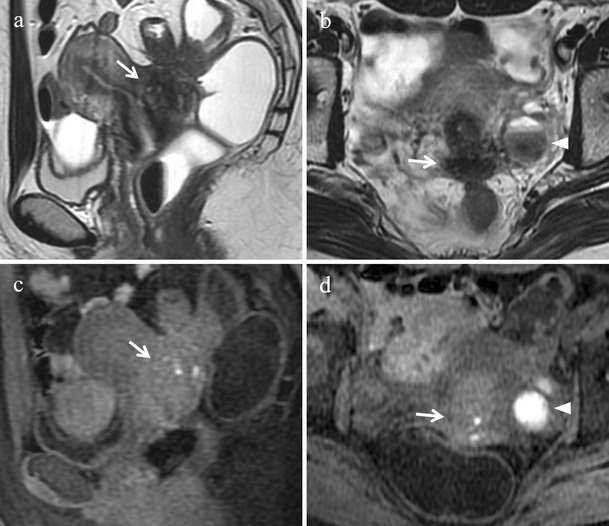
Endometriosis of the retrocervical region in a 41-year-old woman with dysmenorrhea and dyspareunia, who underwent previous laparoscopic adhesiolysis. (a) Sagittal and (b) oblique axial T2-weighted images show a hypointense ill-defined infiltrative tissue (white arrows) involving the posterior portion of the cervix, the retrocervical region and the anterior rectal wall. Small cystic cavities are seen within the lesion. On (c) sagittal and (d) axial fat-suppressed T1-weighted images the lesion exhibits intermediate signal intensity (white arrows) with small hyperintense foci reflecting haemorrhage. Note the small left hemorrhagic cyst (white arrowheads in b and d) showing a fluid-fluid level on T2-weighted image; it disappeared in the follow-up US examinations and was likely a hemorrhagic follicular cyst
